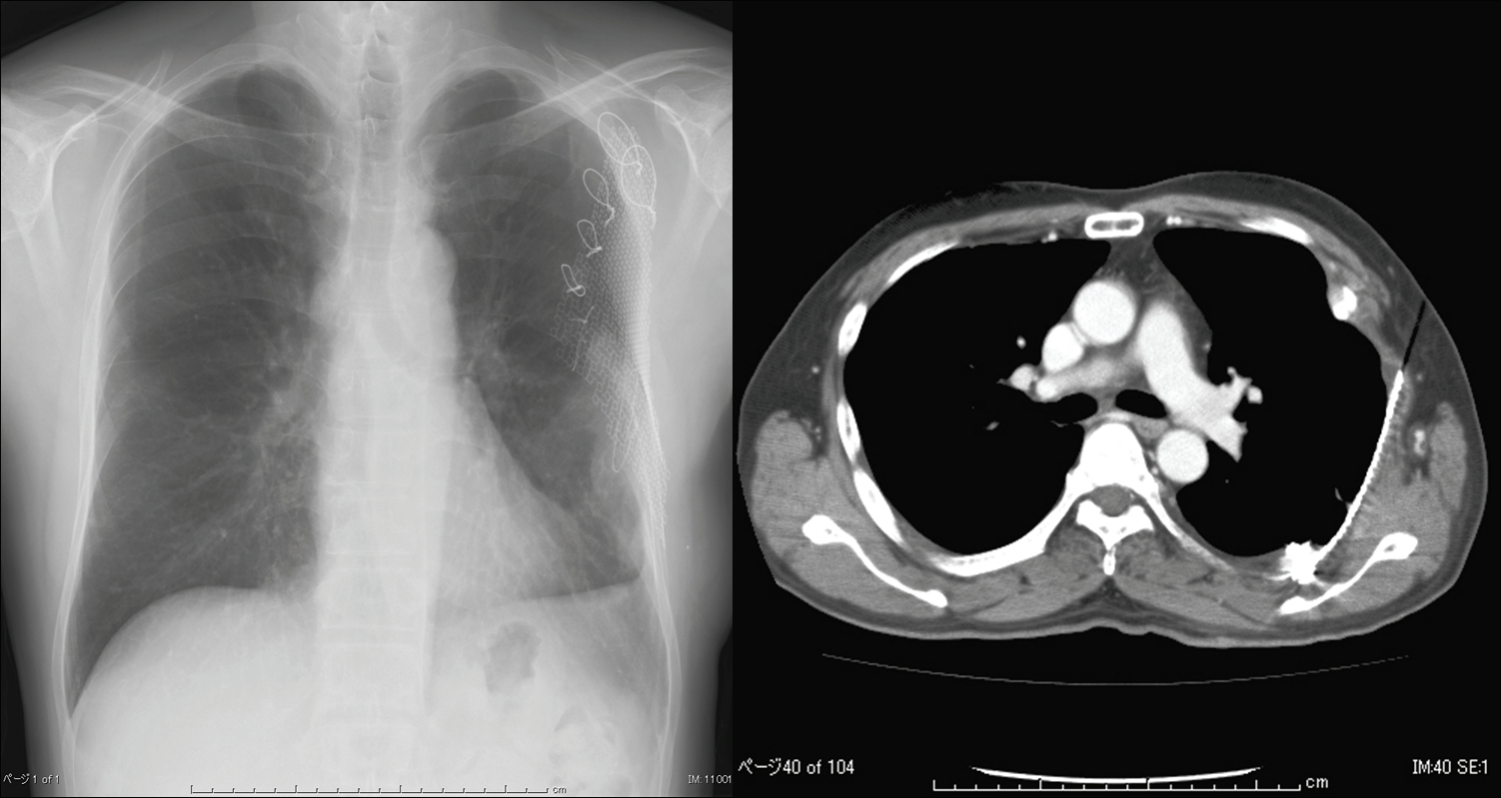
Chest X-ray and computed tomography scan after surgery. Chest X-ray and computed tomography scan showed that the left lung had completely expanded and reconstruction of the thoracic defect was successfully achieved.
